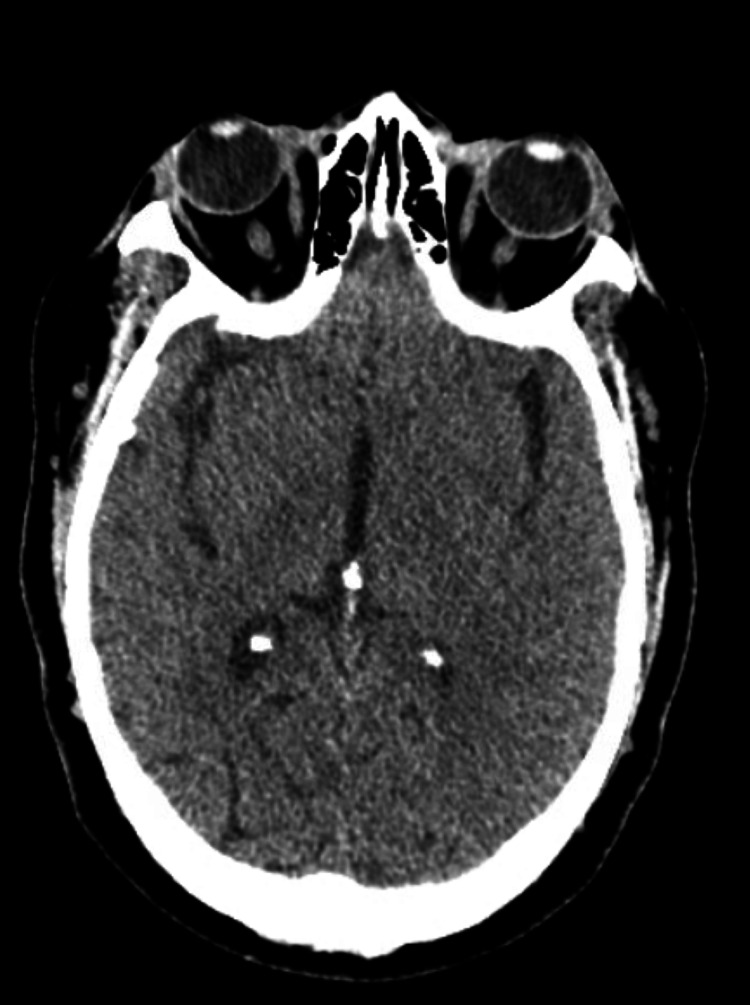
Non-contrast CT of the head. Non-contrast CT of the head showing no acute intracranial abnormalities. No acute intracranial hemorrhage or displaced cranial fracture.
